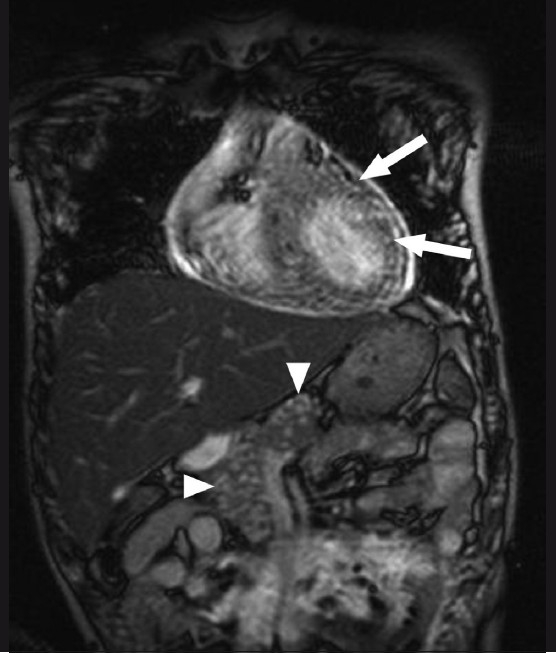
Balanced gradient MRI coronal image of the chest and upper abdomen shows hyperintense nodular lesions in the cardiac muscles (arrows) and pancreas (arrowhead)
